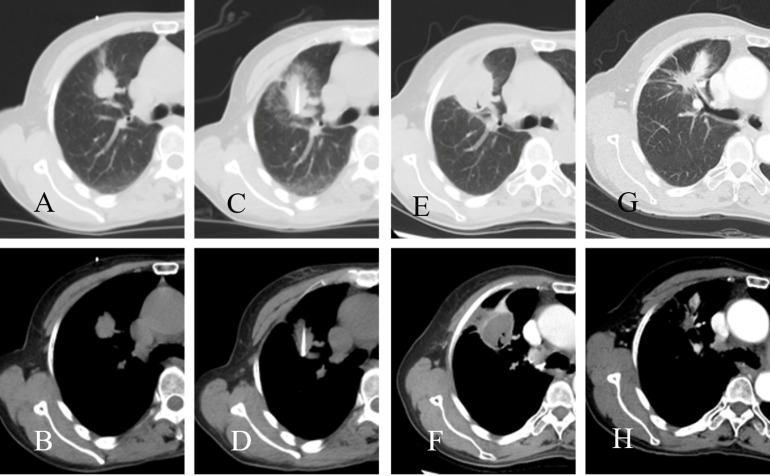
CT images of a patient who underwent radiofrequency ablation A., B. before radiofrequency ablation; C., D. during radiofrequency ablation E., F.; three months after radiofrequency ablation; G., H. 24 months after radiofrequency ablation.
